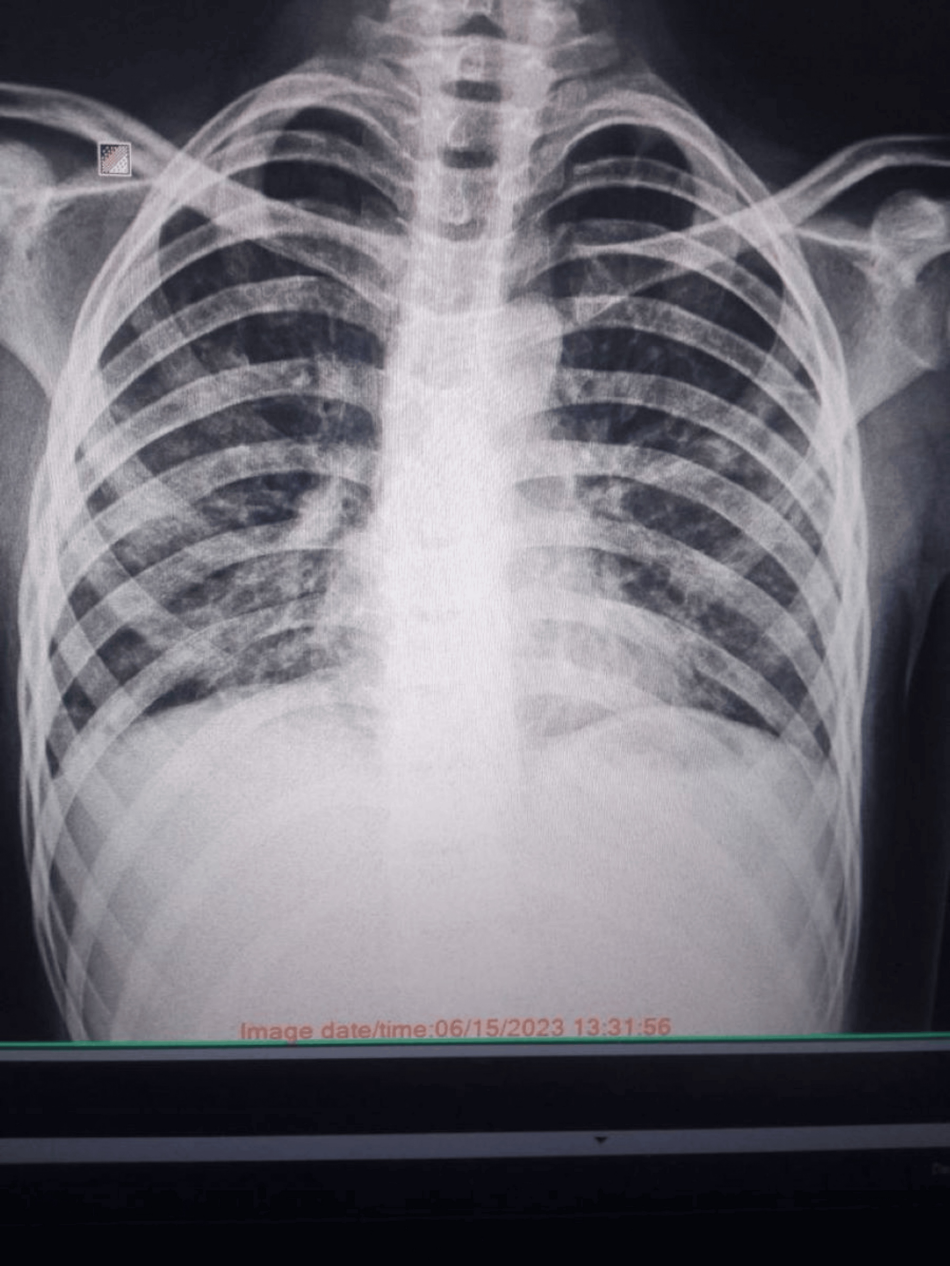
Chest X-ray on a follow-up visit
